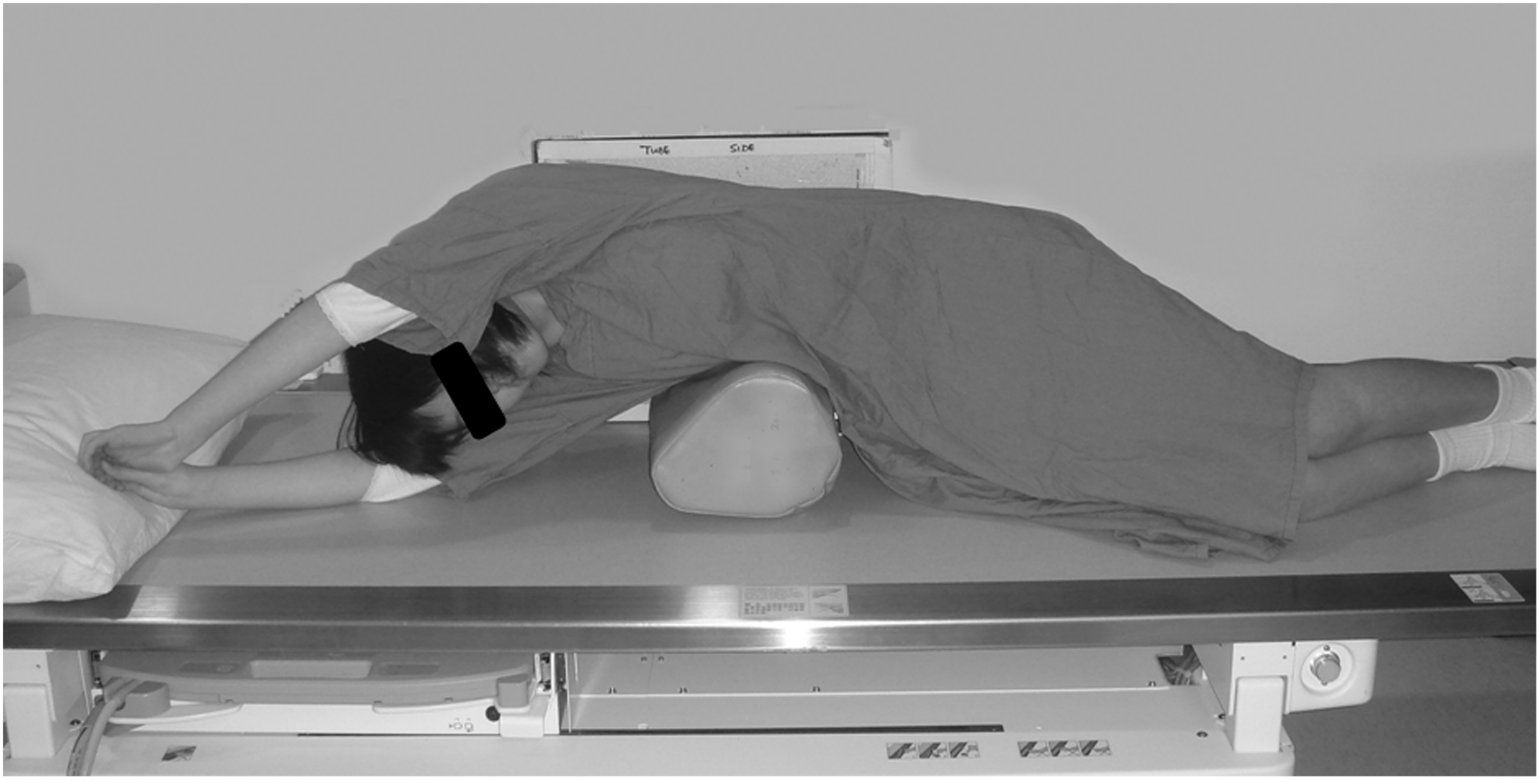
Fulcrum bending radiograph. The patient is positioned on the lateral decubitus position. A padded cylinder (fulcrum) of appropriate size is placed on the side of the curve at the level of the rib corresponding to the apex of the curve. For example, if the apex vertebra of the curve is at T9, the fulcrum should be placed at the T9 rib. The fulcrum should be positioned to allow the shoulder and the pelvis to be lifted off the table.
